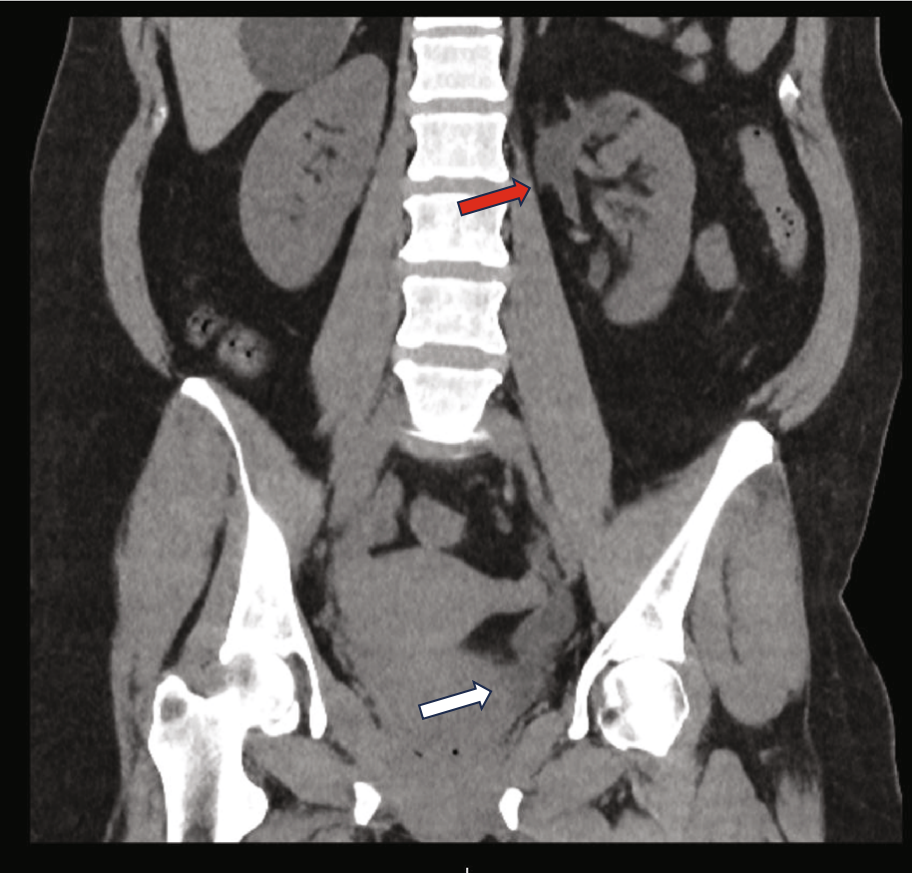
CT urinary tract, 1 year postoperatively: coronal section. Resolving upper moiety dilatation (red arrow). No evidence of the previous calculus (white arrow).
